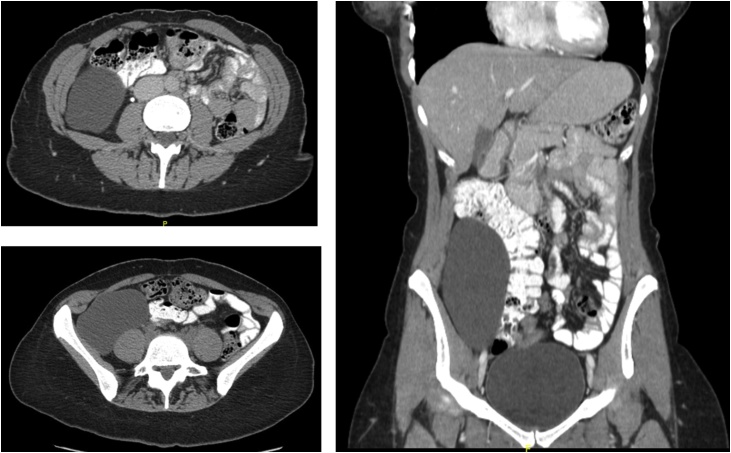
Contrast-enhanced CT abdomen showing a huge uni-loculated mass in the right side lying over the paravertebral muscles and displacing the right colon and whole bowel to the left side. The mass is extending in right para-colic gutter from hepatic flexure down to the uterus.
